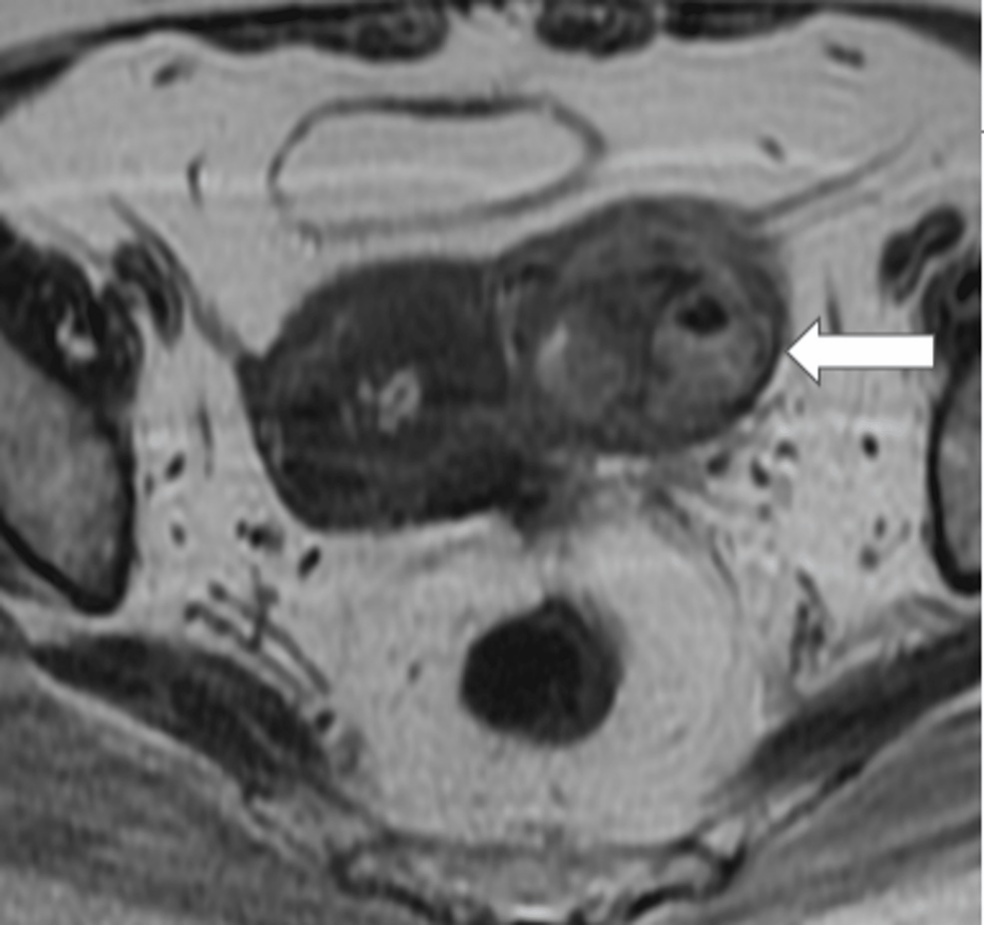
MRI coronal T2-weighted image showing unilateral adnexal lesion on left side.
